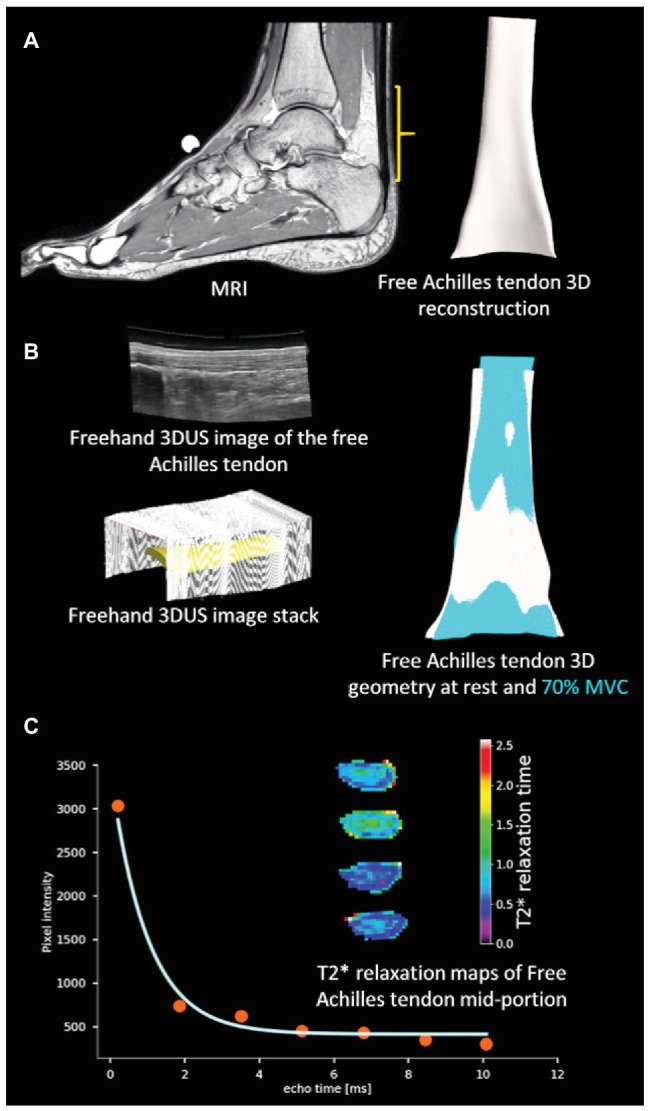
Overview of methodology: (A) three-dimensional (3D) reconstruction of the free Achilles tendon from MRI (B) 3D ultrasound (3DUS) reconstruction of free Achilles tendon from freehand 3DUS images and free Achilles tendon geometry during rest and active muscle contraction condition [70% maximal voluntary contraction (MVC)], and (C) T2^*^ relaxation time of a sample pixel in the free Achilles tendon by fitting an exponentially decaying curve, and sample colour maps of image slices of the free Achilles tendon mid-portion.
